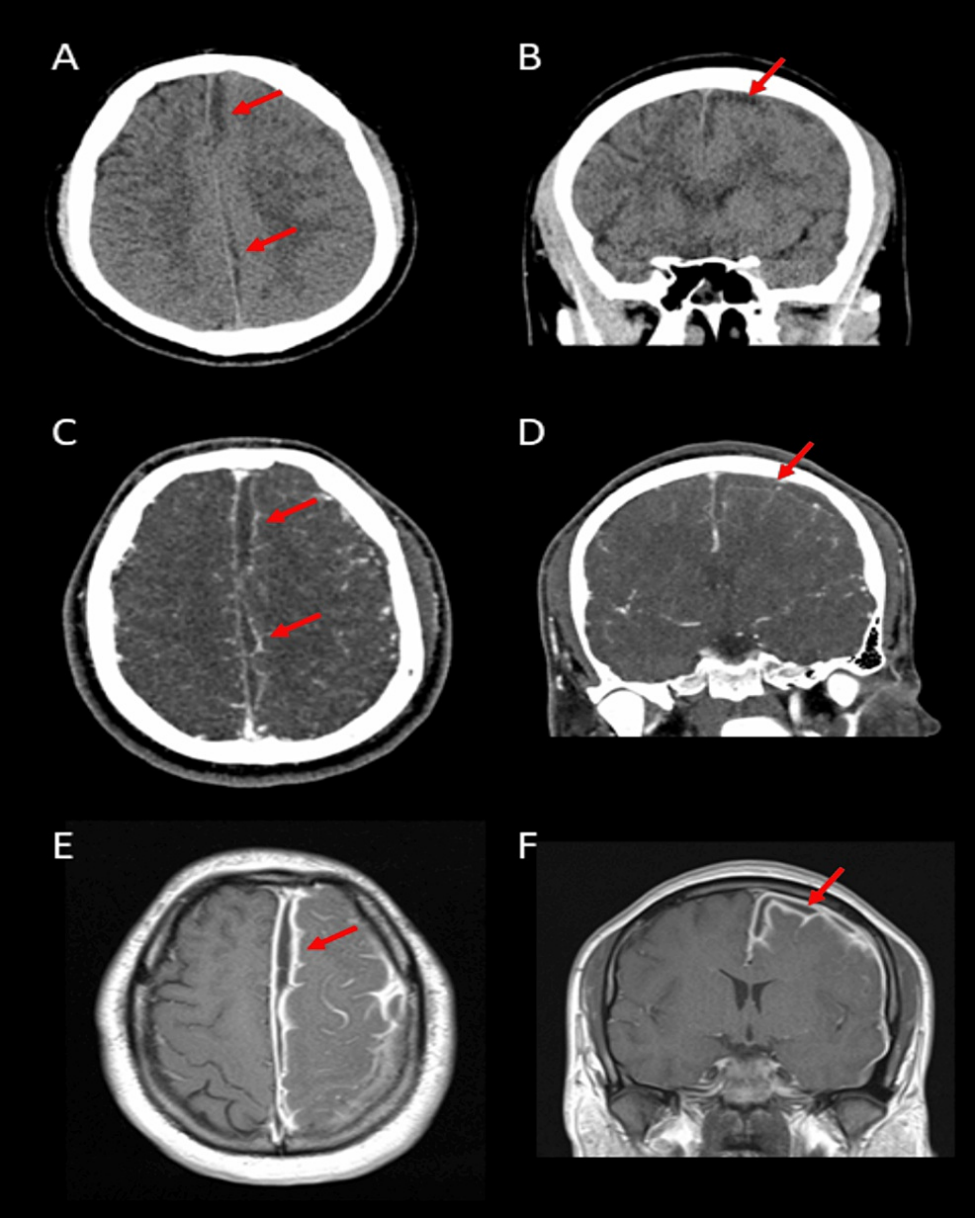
Imaging of the subdural empyema Head CT without contrast (A, B) demonstrates the subdural empyema (red arrows) along the falx cerebri and cerebral convexity on the left. Axial (C) and coronal (D) views of the head CT angiogram show the left subdural empyema (red arrows) with the absence of contrast extravasation. Post-contrast T1-weighted brain MRI (E, F) demonstrates diffuse enhancement of the meninges around the left subdural empyema (red arrows).
